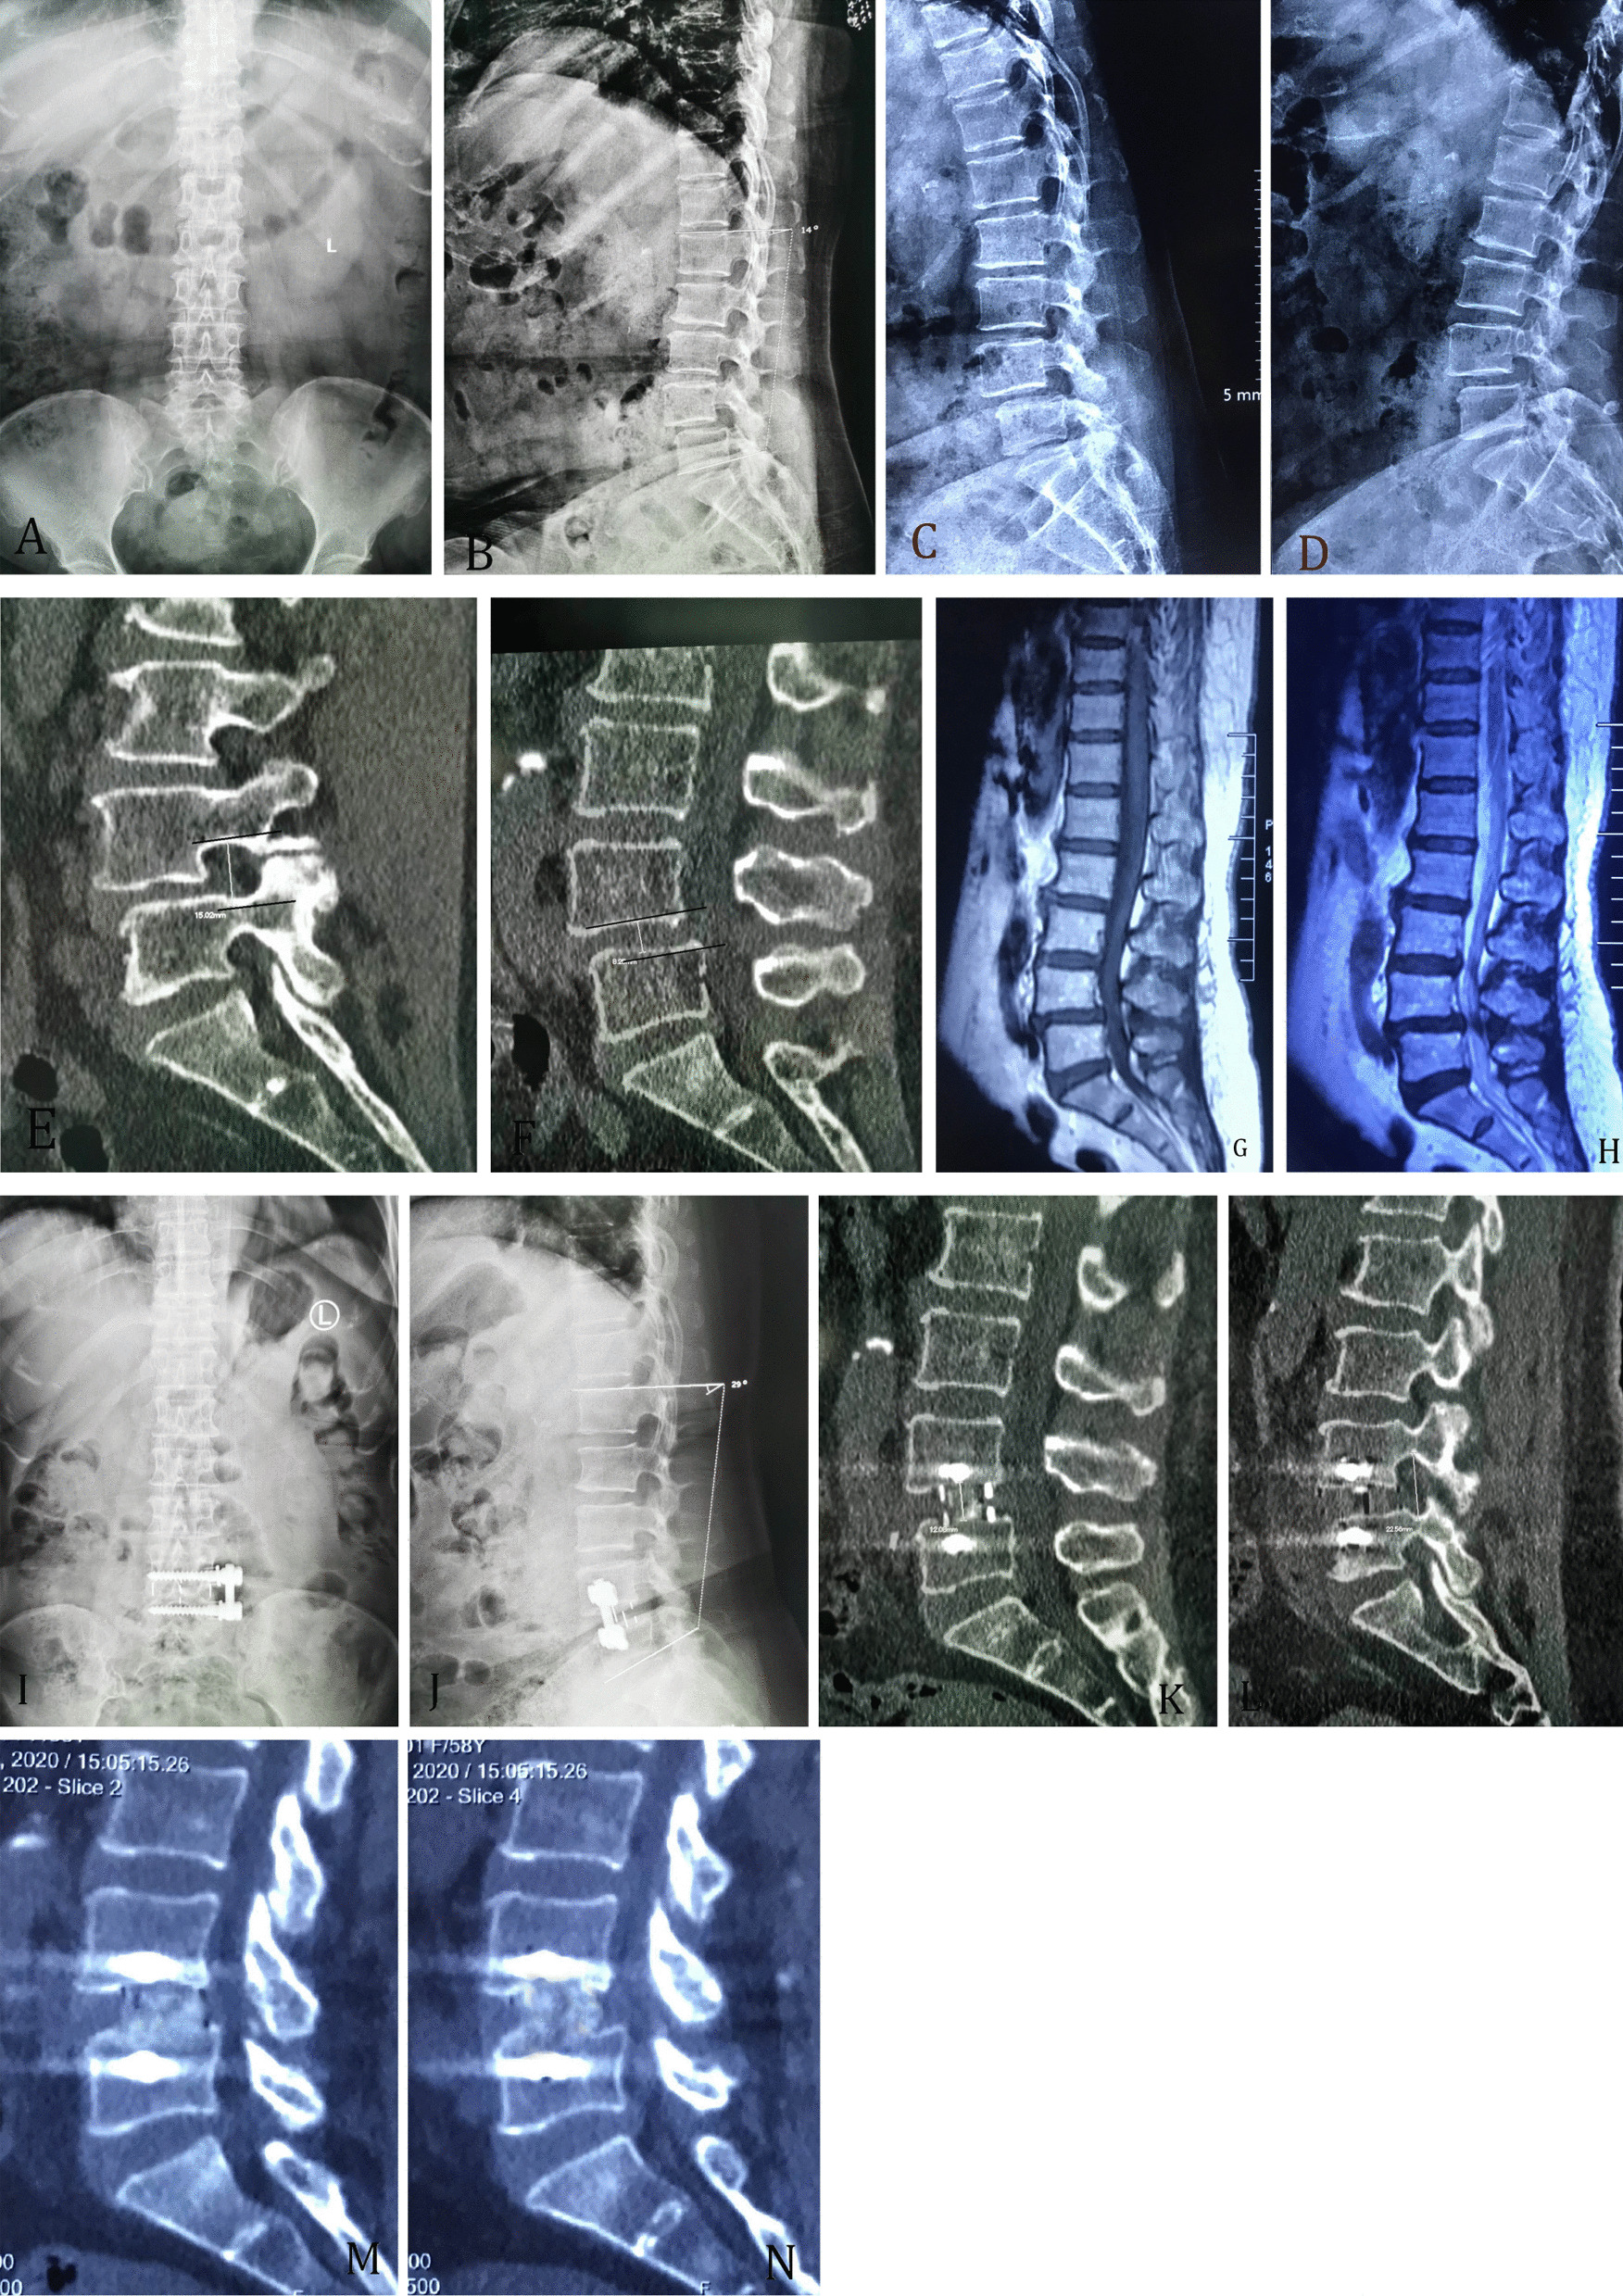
A typical case. A–D Pre-operative radiographies with lordosis of 14°; E height of intervertebral foramen before surgery; F height of intervertebral space before surgery; G pre-operative MRI T1 weighted imaging H,pre-operative MRI T2 weighted imaging; I, J post-operative radiographies with lordosis of 29°; K post-operative intervertebral space height; L post-operative intervertebral foramina height; M 12 months post-surgery,solid fusion at upper endplate of L4. N 24 months post-surgery solid fusion at the upper and lower endplates
